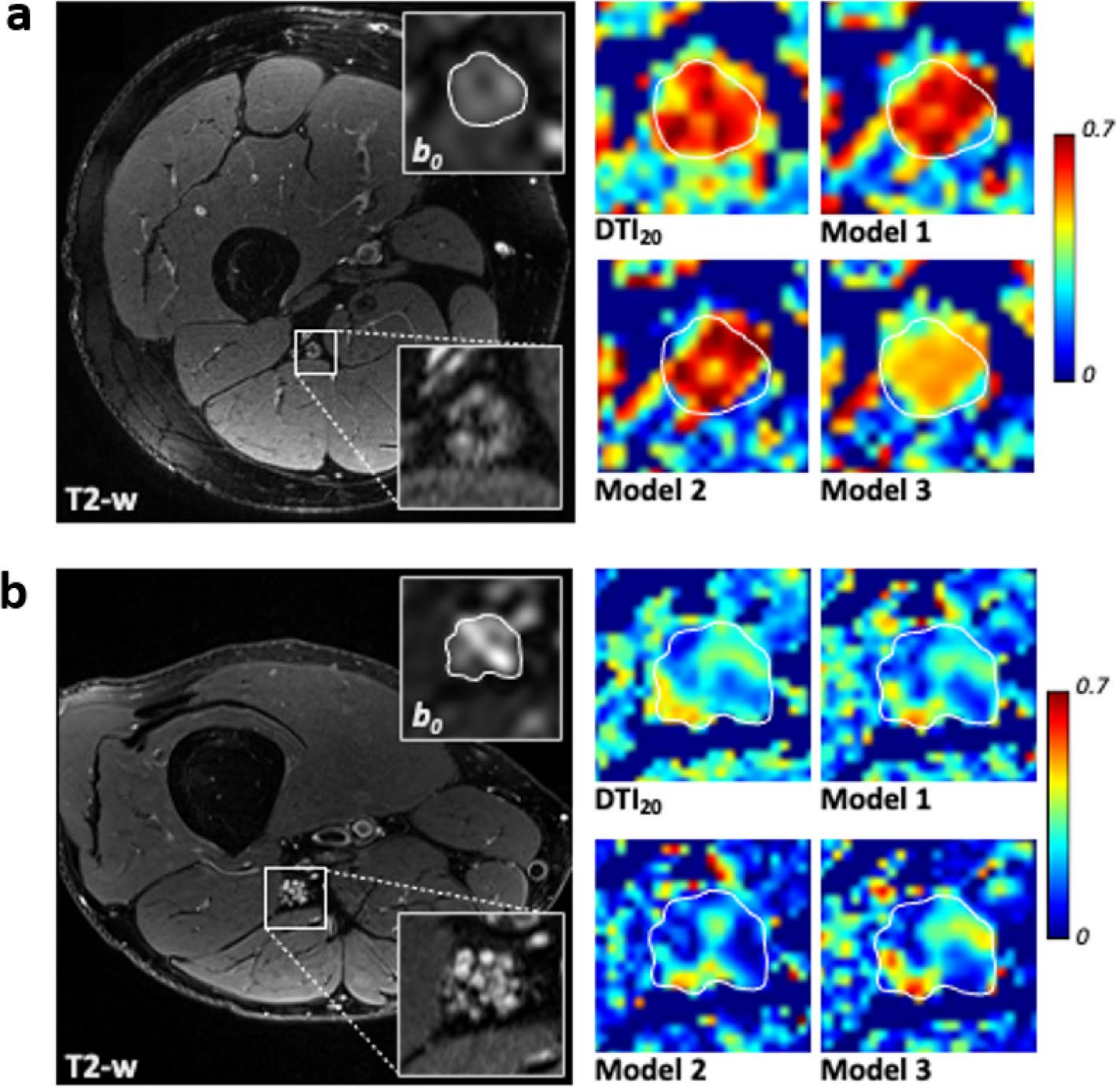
Fractional anisotropy (FA) map reconstructions in healthy and diabetic patients. Representative images of a healthy subject (a) and a diabetic patient (b) showing T2-weighted images of the distal sciatic nerve and corresponding color-coded fractional anisotropy (FA) maps calculated with the 20-directional standard model (DTI_20_) and the reduced models 1, 2, and 3. Segmentation of the nerve portion is indicated by a white contour. Insets show a magnification of the nerve. Model 1 achieves the best approximation to DTI_20_ in both healthy and diseased nerve tissue, while models 2 and 3 yield good but inferior accuracy
